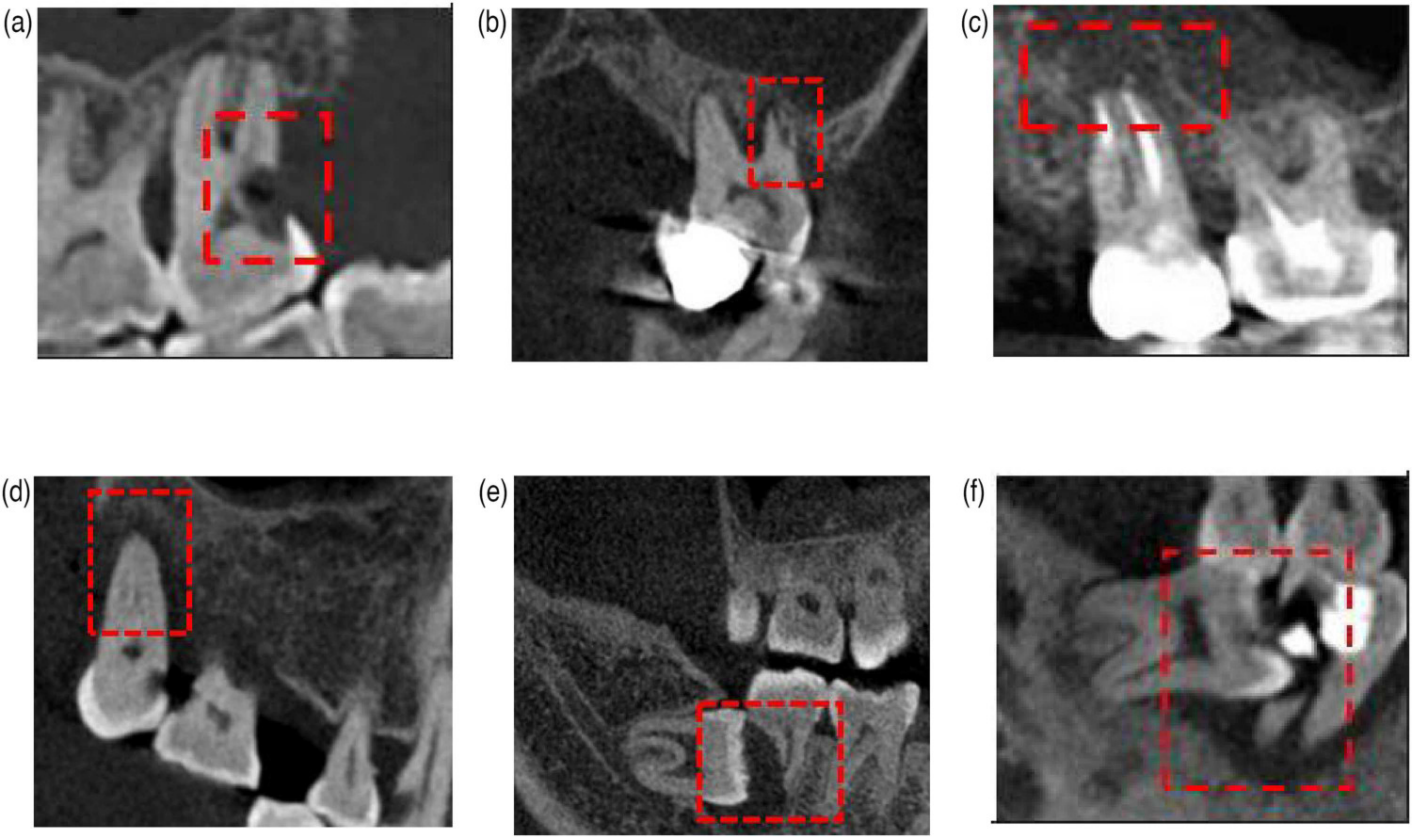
Reasons for second molar extractions. (a) Endodontic factors: severe caries. (b) Endodontic factors: cracked teeth. (c) Endodontic factors: periapical lesions. (d) Periodontal factors: severe periodontitis. (e) Impacted third molar-related factors. (f) Impacted third molar-related factors.
